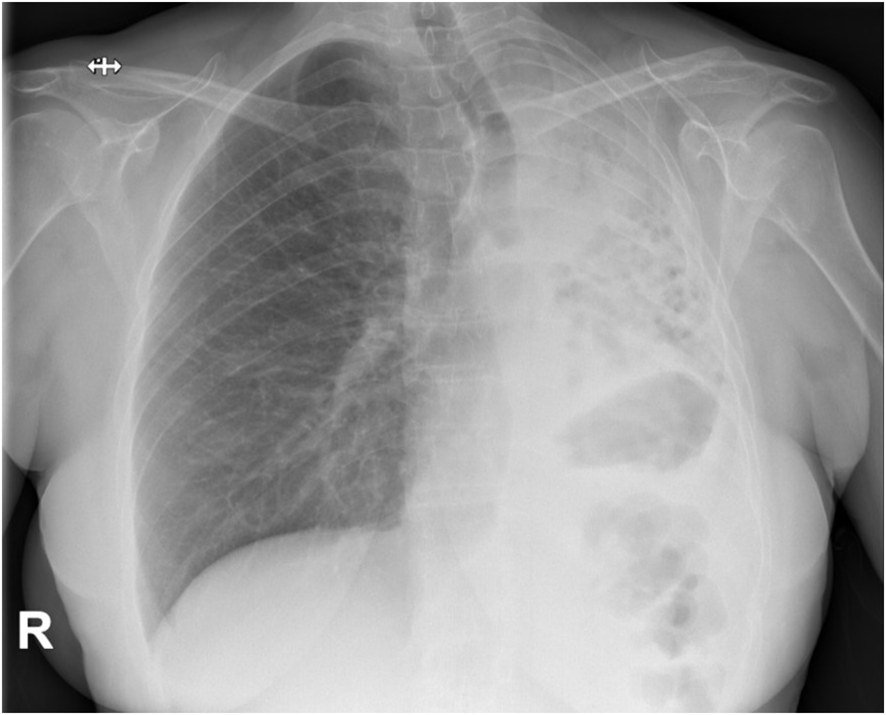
Chest X-ray of the patient at the emergency department with complete atelectasis of left lung.
